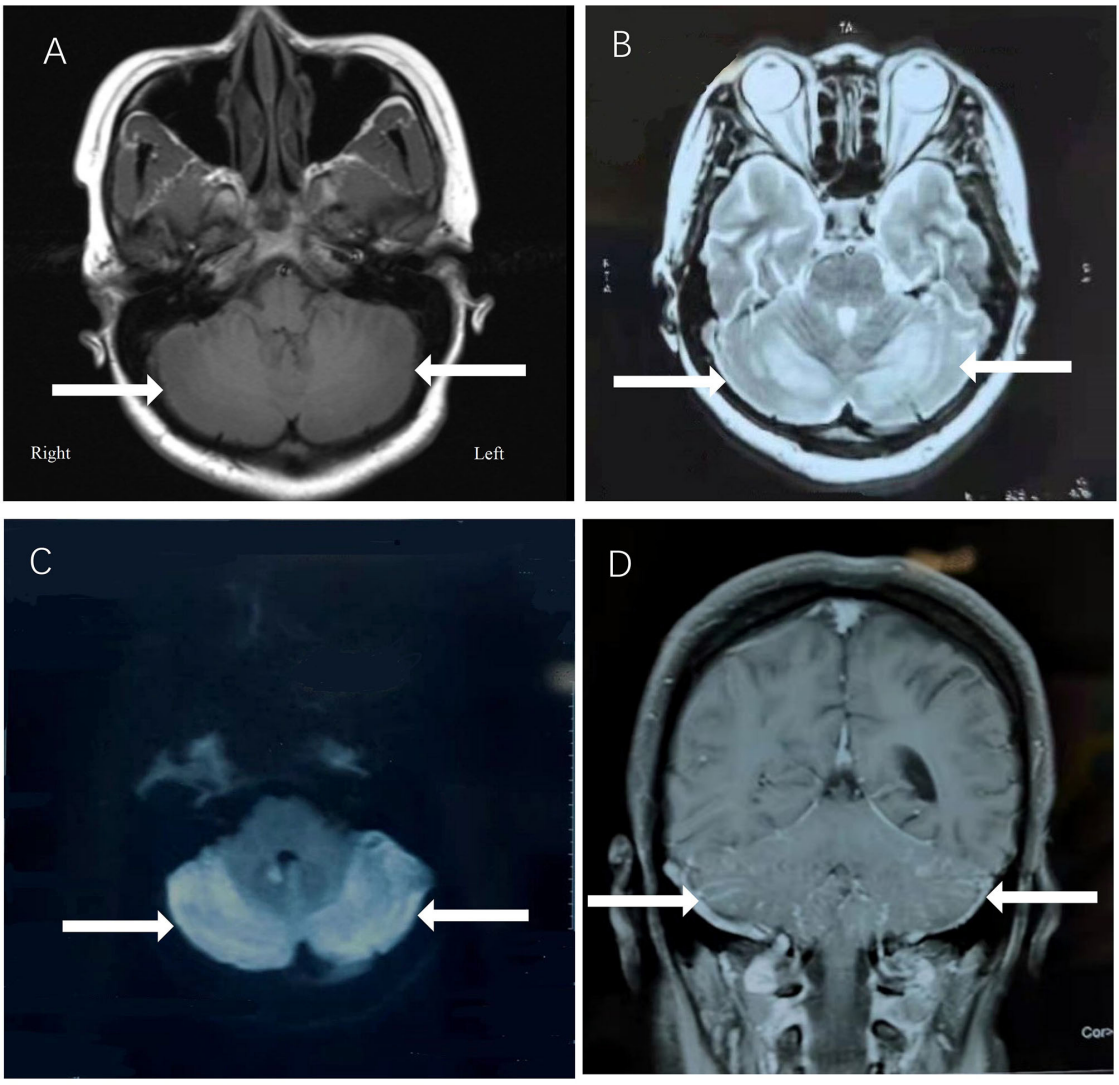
The patient's brain MRI showed abnormal signals in the vermis and in the bilateral cerebellar hemispheres, with low signal on T1-weighted image (A), high signal on T2-weighted image (B), and high signal on DWI sequence (C) with Gd enhancement (D).
